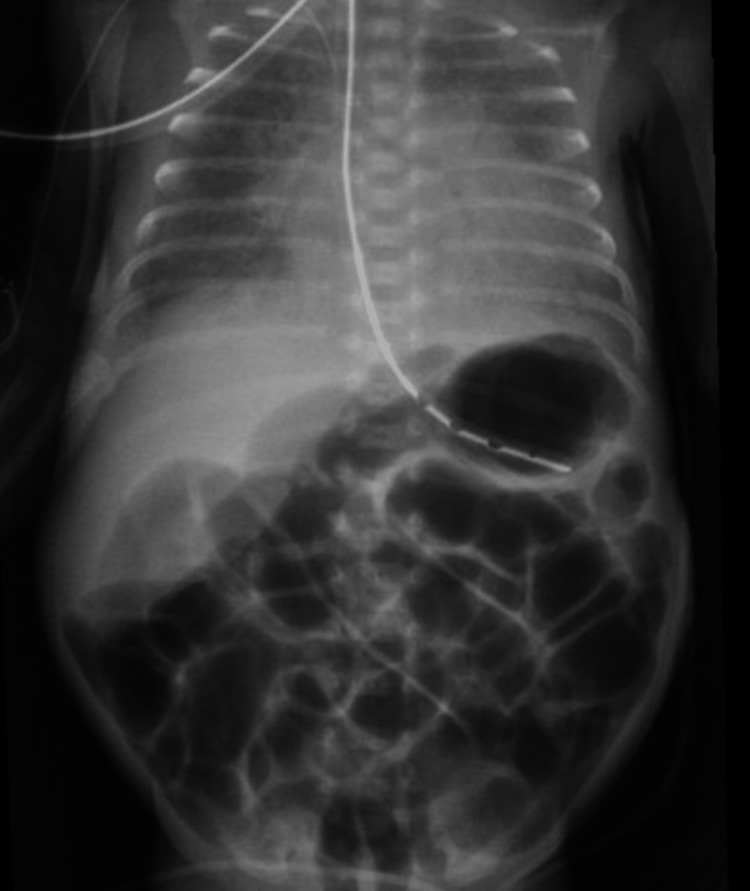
KUB radiograph showing worsening gaseous distension of the stomach and small intestine, without evidence of free intraperitoneal air or pneumatosis in the small intestinal wall KUB: kidney, ureter, and bladder
